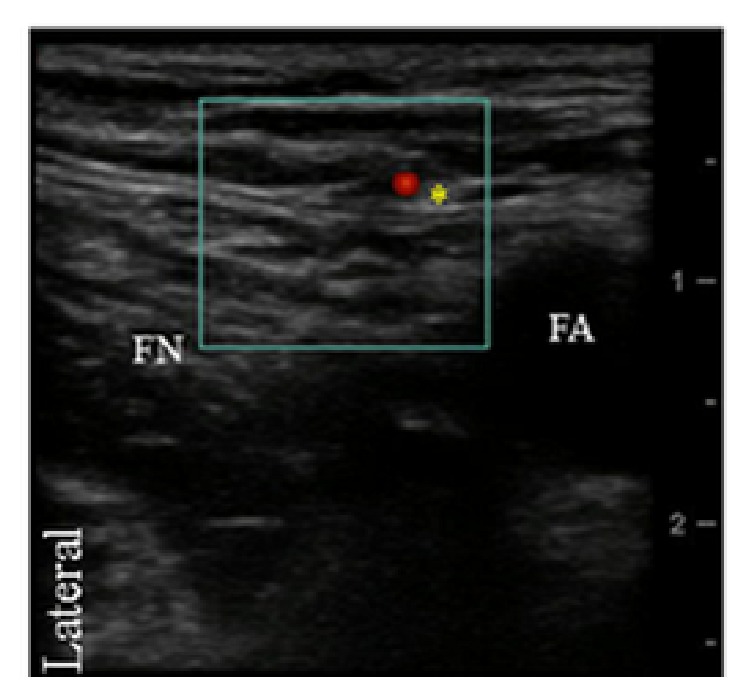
Transverse ultrasound image of the femoral nerve (FN) and femoral artery (FA). The anterior femoral cutaneous nerve of the thigh with its accompanying artery is also shown (asterisk).
